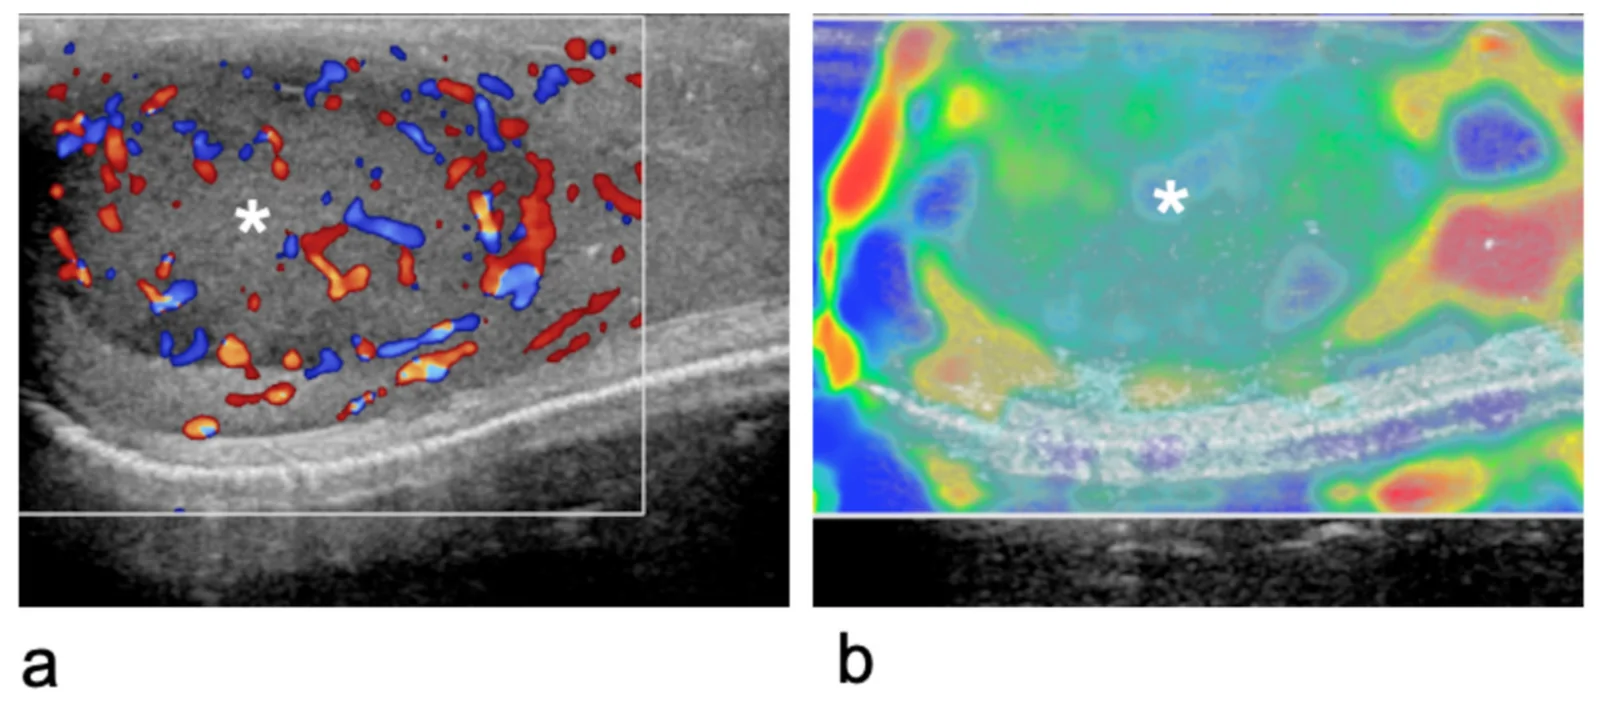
A 26-year-old man was referred for painful enlargement of the right testis that had developed after minor scrotal trauma three weeks earlier. In the preceding days, he had noticed a reduction in the size of the right hemiscrotum. He denied fever, and no palpable lesion was detected. Ultrasound shows a testicular lesion (asterisk) that is markedly vascular on colour Doppler imaging (a) and soft on elastography (b). At surgery, the lesion proved to be a seminoma with intralesional haemorrhage, likely secondary to the preceding trauma.
